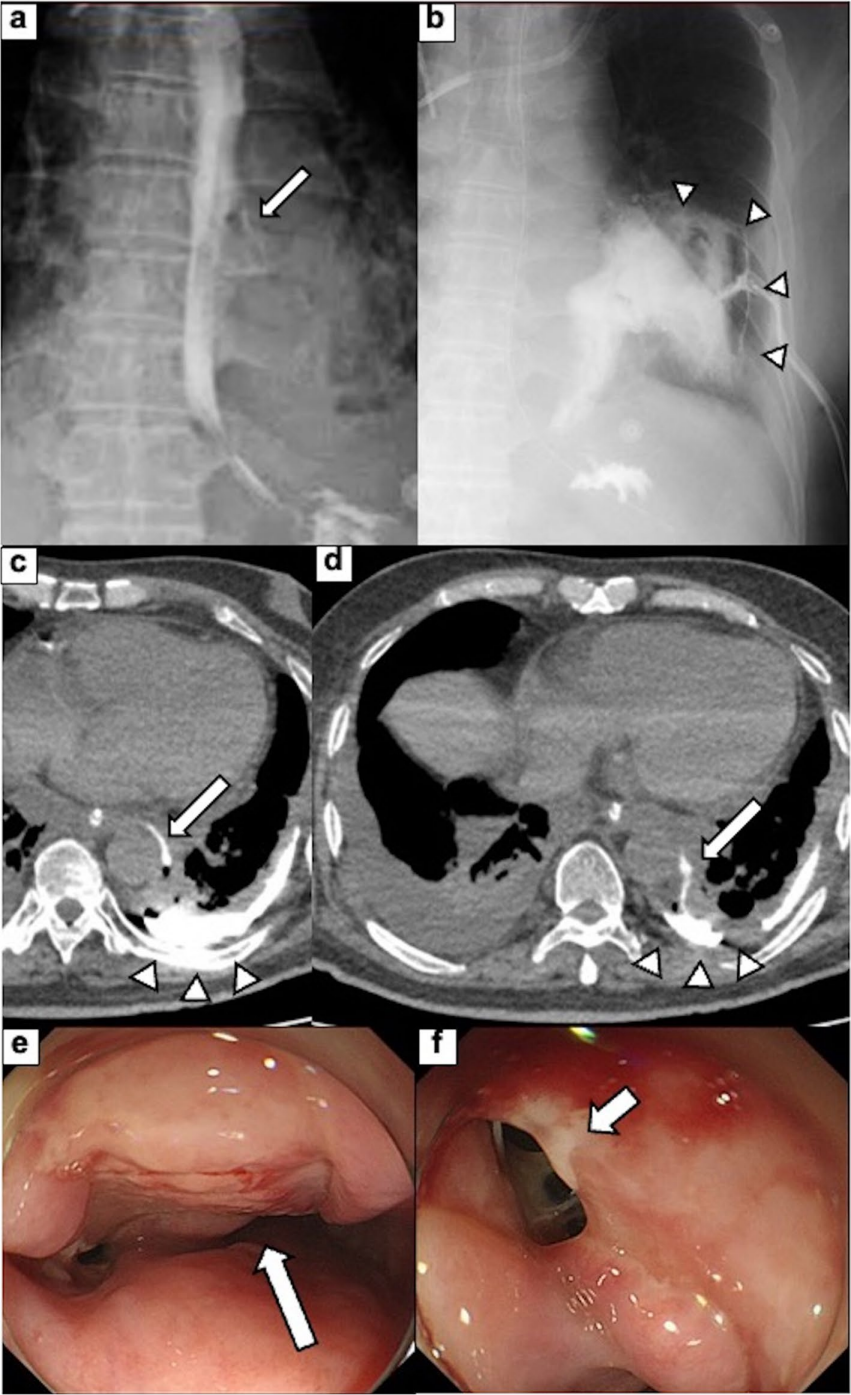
a, b Upper gastrointestinal radiography and (c, d) CT on hospital day 22. The findings revealed that there is leakage of the contrast from the esophagus to the left pleural cavity (a, c, and d, white arrow) and pleural effusion in bilateral thoracic cavities b, c, and d, white arrowhead). e, f EGD finding; the ulcer around the perforation (e, white arrow) and the perforation on the esophageal wall (f, white arrow)
